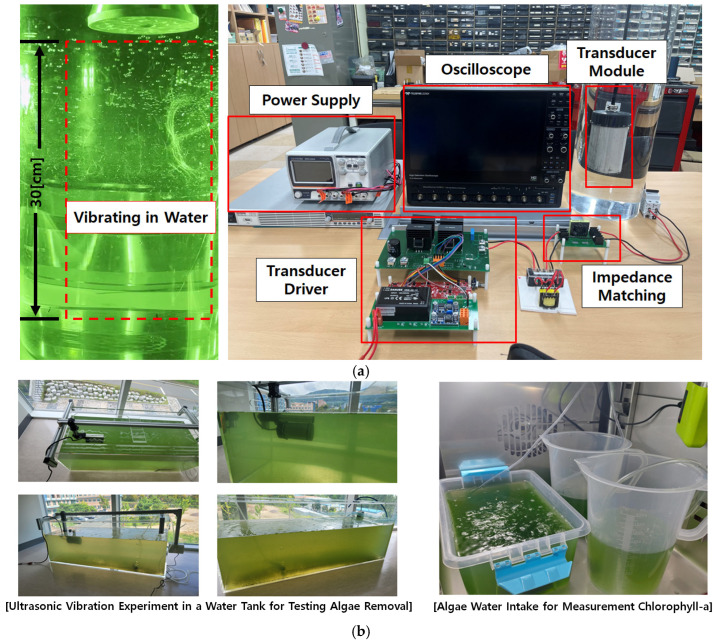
(a) Experimental, hardware configuration, and experiment result of transducer vibrating in water; (b) ultrasonic vibration experiment in water tank and algae water intake for measurement chlorophyll-a.
